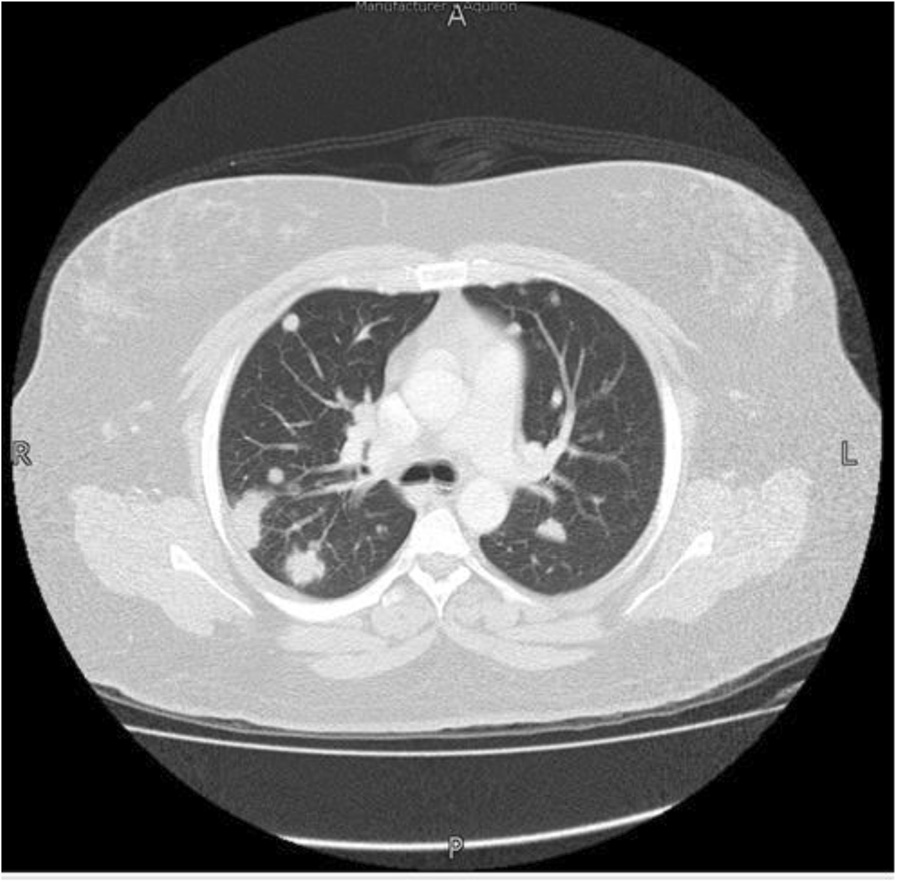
CT chest of Case 1: This is the CT scan of the same 32 year- old female who had presented with a 2-month history of fever, dry cough, exertional dyspnea and weight loss. She was treated with a number of antibiotics with no significant improvement. This scan further elaborated on the diffuse bilateral pulmonary nodular infiltrates which were evident on the chest radiograph shown in Figure 1. (A= anterior, L= left, P= posterior, R= right ).
